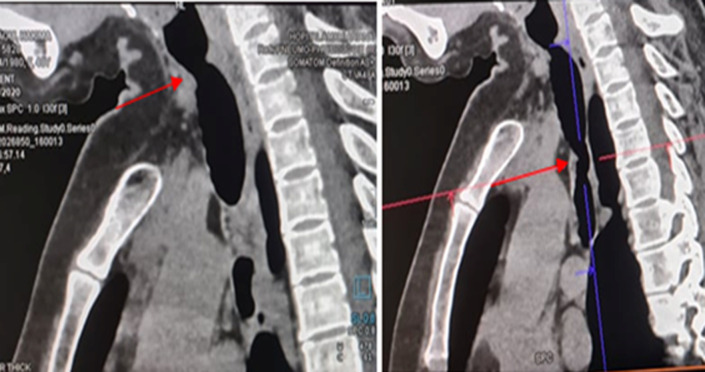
sagittal sections of thoracic computed tomography showing double tracheal narrowing approximately 20 mm below the vocal cords and 40 mm from the carina
